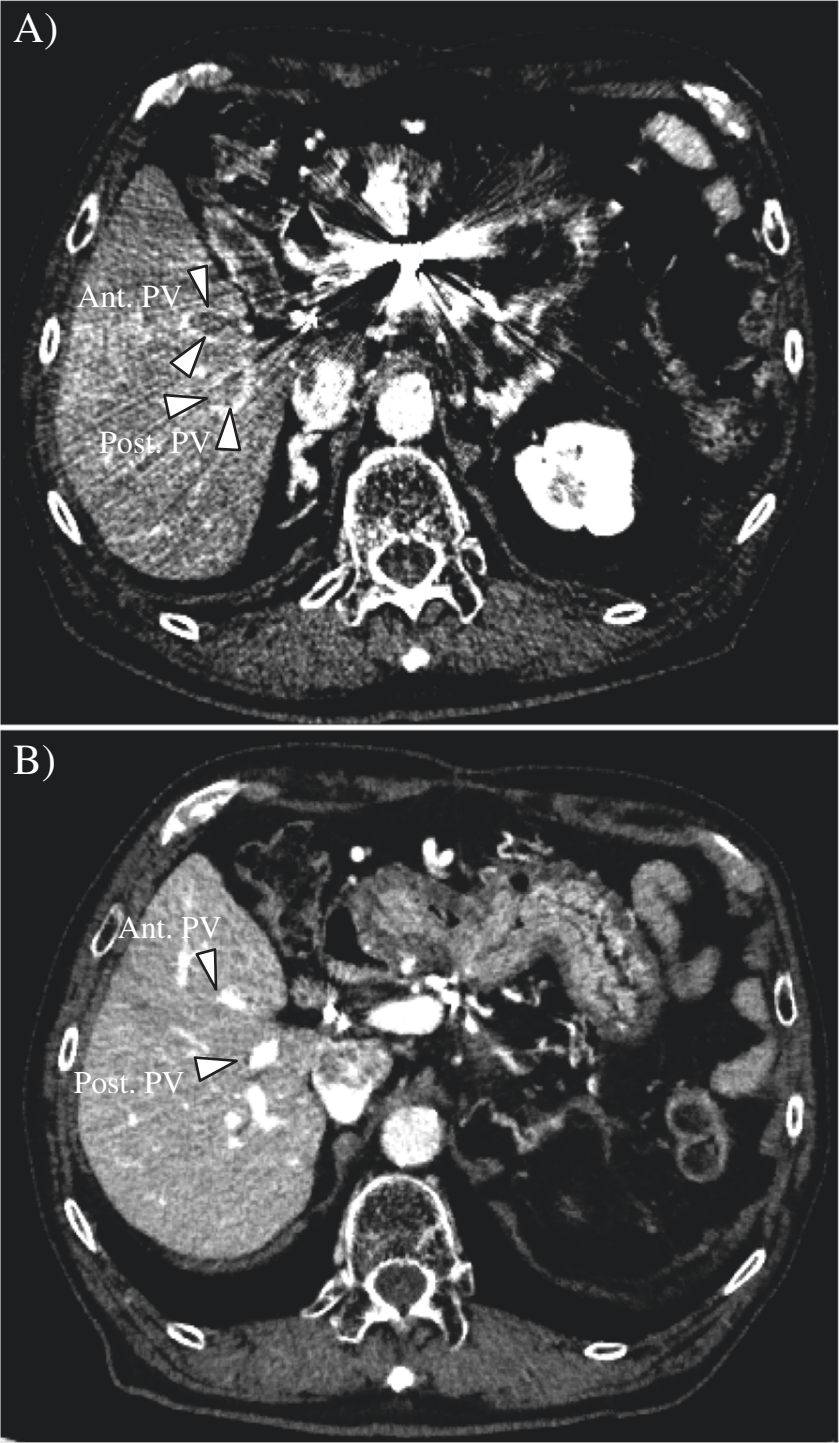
Abdominal contrast-enhanced computed tomography before and after sorafenib introduction. (A)
